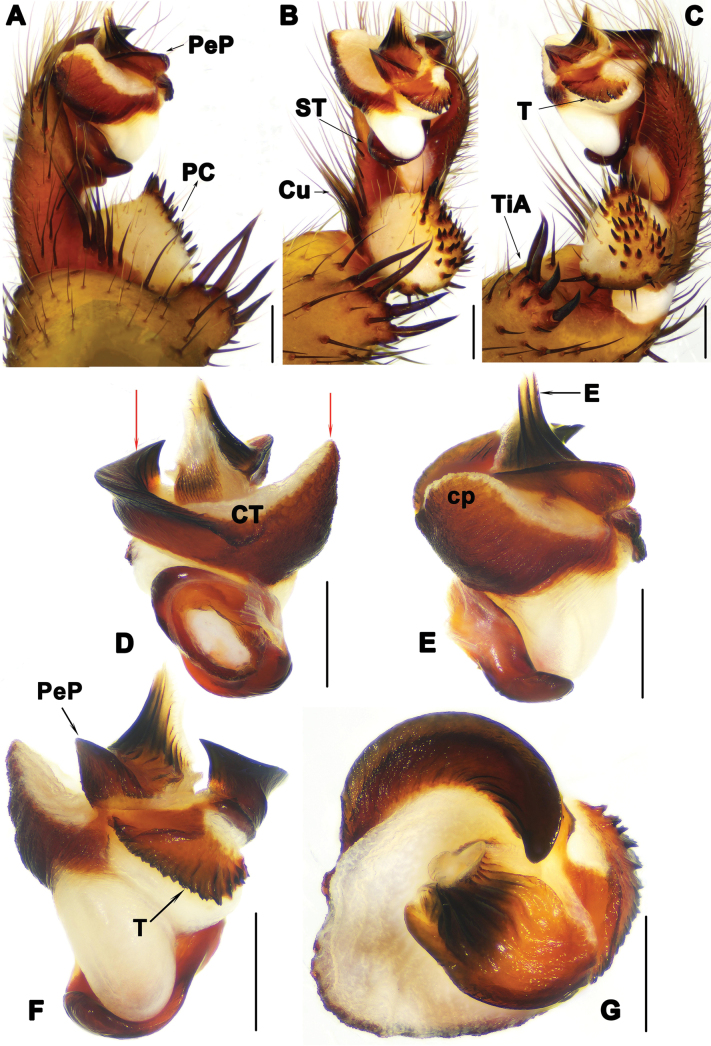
Male palp of *Liphistiusnawngau* sp. nov. A, E prolateral view B ventral view C, F retrolateral view D dorsal view, red arrows show arched projection and triangular process of CTG distal view. Abbreviations: CT = contrategulum; cp = contrategular process; Cu = cumulus; E = embolus; PC = paracymbium; PeP = paraembolic plate; ST = subtegulum; T = tegulum; TiA = tibial apophysis. Scale bars: 0.5 mm.
